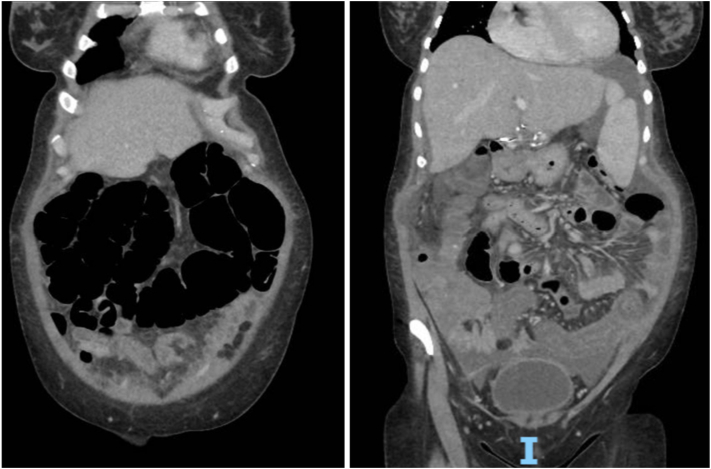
CT images demonstrating severe left-sided colitis, distension of the transverse colon, and fluid-filled distension of the cecum, up to 6 cm, without evidence of obstruction, toxic megacolon, or bowel perforation, and small to moderate volume ascites. CT, computed tomography.
